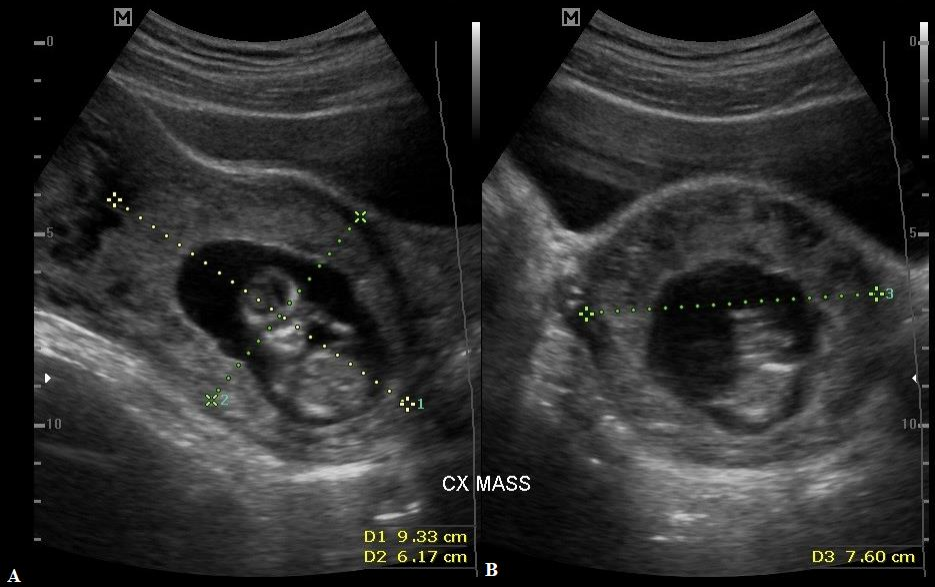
(A,B) transabdominal ultrasound image showing the cervical ectopic gestation measuring 9.33 cm x 7.60 cm x 6.17 cm; the pregnancy is restricted to the endocervical canal
